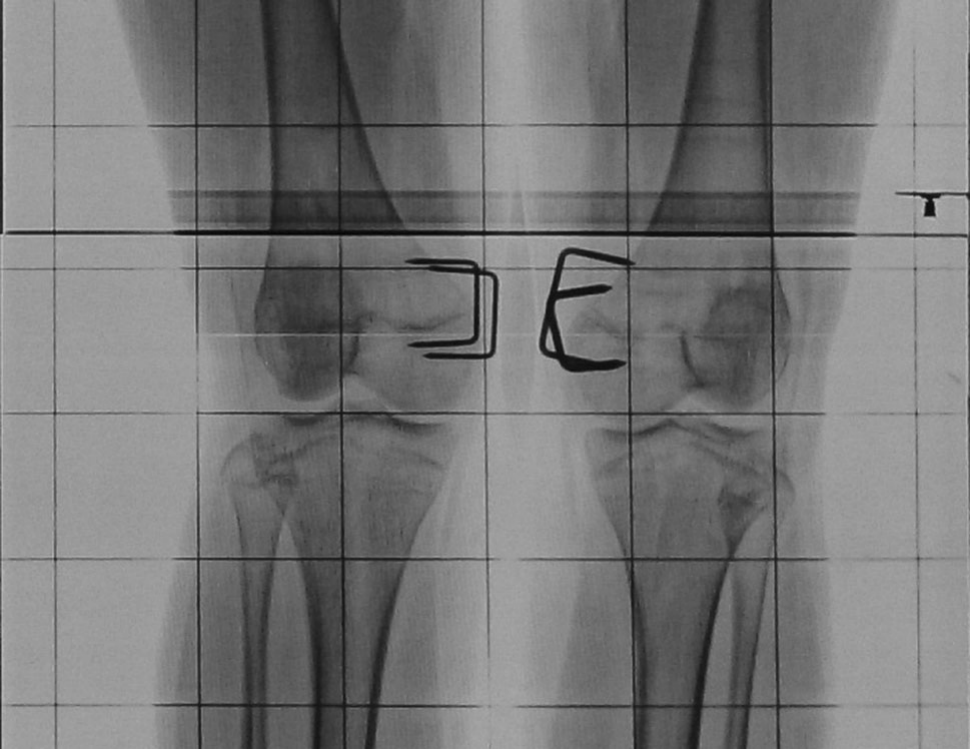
Radiograph showing complication of stapling (migration) 4 months post-operatively
